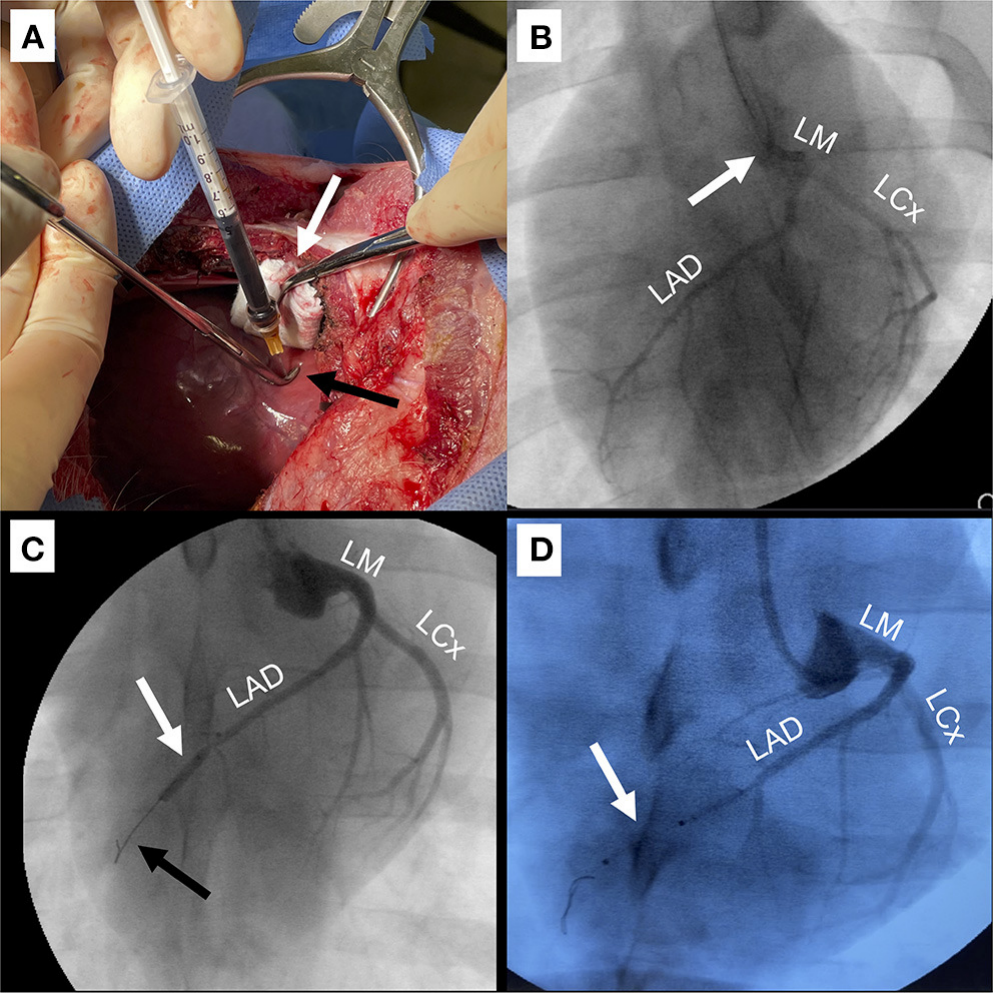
Techniques for delivery of IO/AAV. (A) Intramyocardial injection (IM) through anterior right thoracotomy. The apex was retracted to the right to expose the anterior and apical regions (white arrow) and a 25-gauge 5/8″ needle in a right-angled clamp (black arrow) was used to mediate depth of injection. (B) Direct left main coronary artery infusion (LM). Coronary angiographic catheter (white arrow) was positioned in the LM. Infused radiographic contrast marks targeted coronary arteries including the left circumflex (LCx) and left anterior descending (LAD). (C) Balloon occlusion with distal infusion (BO). Angioplasty balloon was positioned in the mid-LAD (white arrow) and inflated for 60–90 s to occlude distal flow (black arrow), during which IO/AAV was infused through the distal tip of the balloon catheter. (D) Perforated sidewall balloon infusion (SW). IO/AAV was infused through the sidewall of a non-occlusive perforated balloon (white arrow) positioned to extend from the mid- to distal-LAD.
